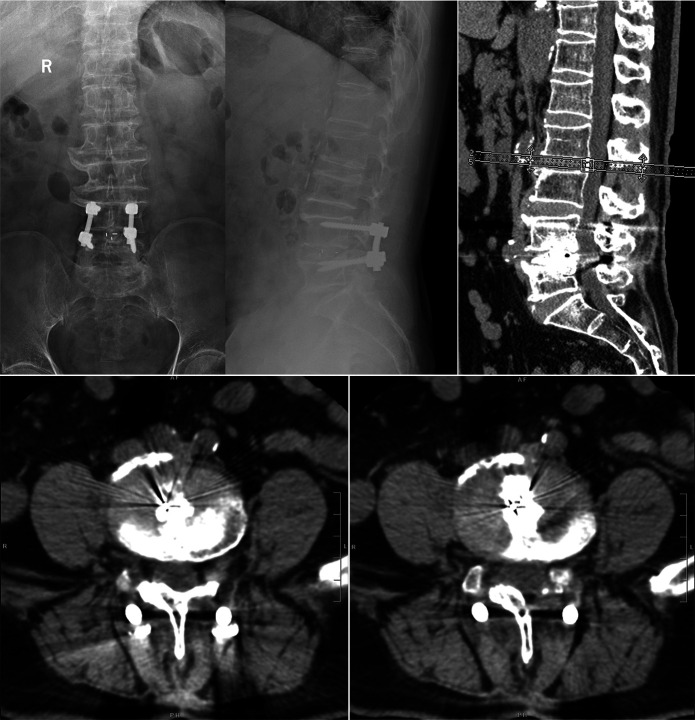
Three-year postoperative follow-up x-ray and CT imaging. At the 3-year postoperative follow-up, radiographic and CT examinations revealed the formation of continuous bony bridges in the grafted region, with no evidence of bony gaps or pseudarthrosis, confirming successful bone fusion. The internal fixation devices, including screws, rods, and interbody fusion cages, were intact and maintained in their normal positions, with no signs of displacement, loosening, or breakage.
